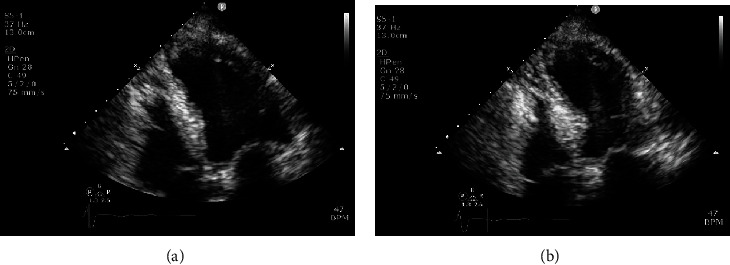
Transthoracic echocardiogram performed on presentation. Apical 4 chamber view, at end diastole (a) and end systolic (b) demonstrating left ventricular apical hypokinesis.
